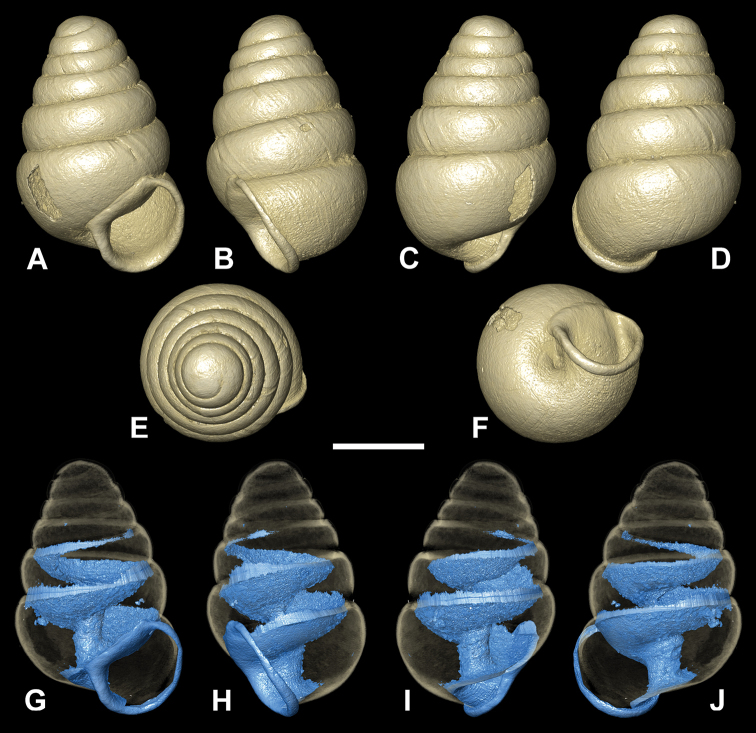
CT scans of *Zospeumgittenbergeri* sp. n. holotype (RMNH.MOL.234166). Scale bar: 500 µm.
